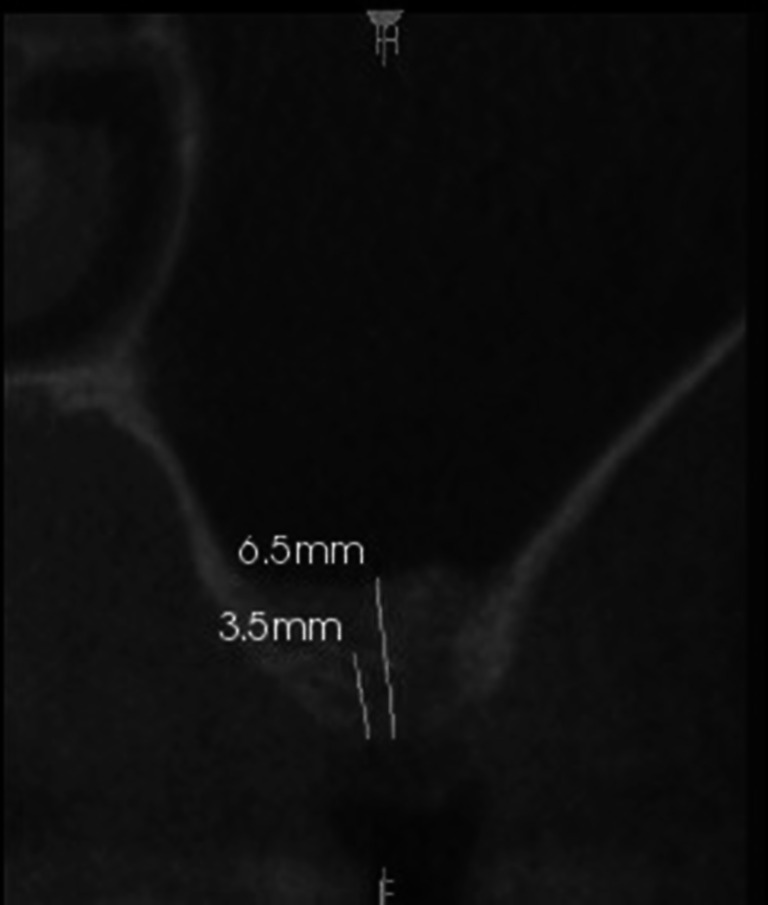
CT scan after stage 1 of the two-stage closed sinus lift
